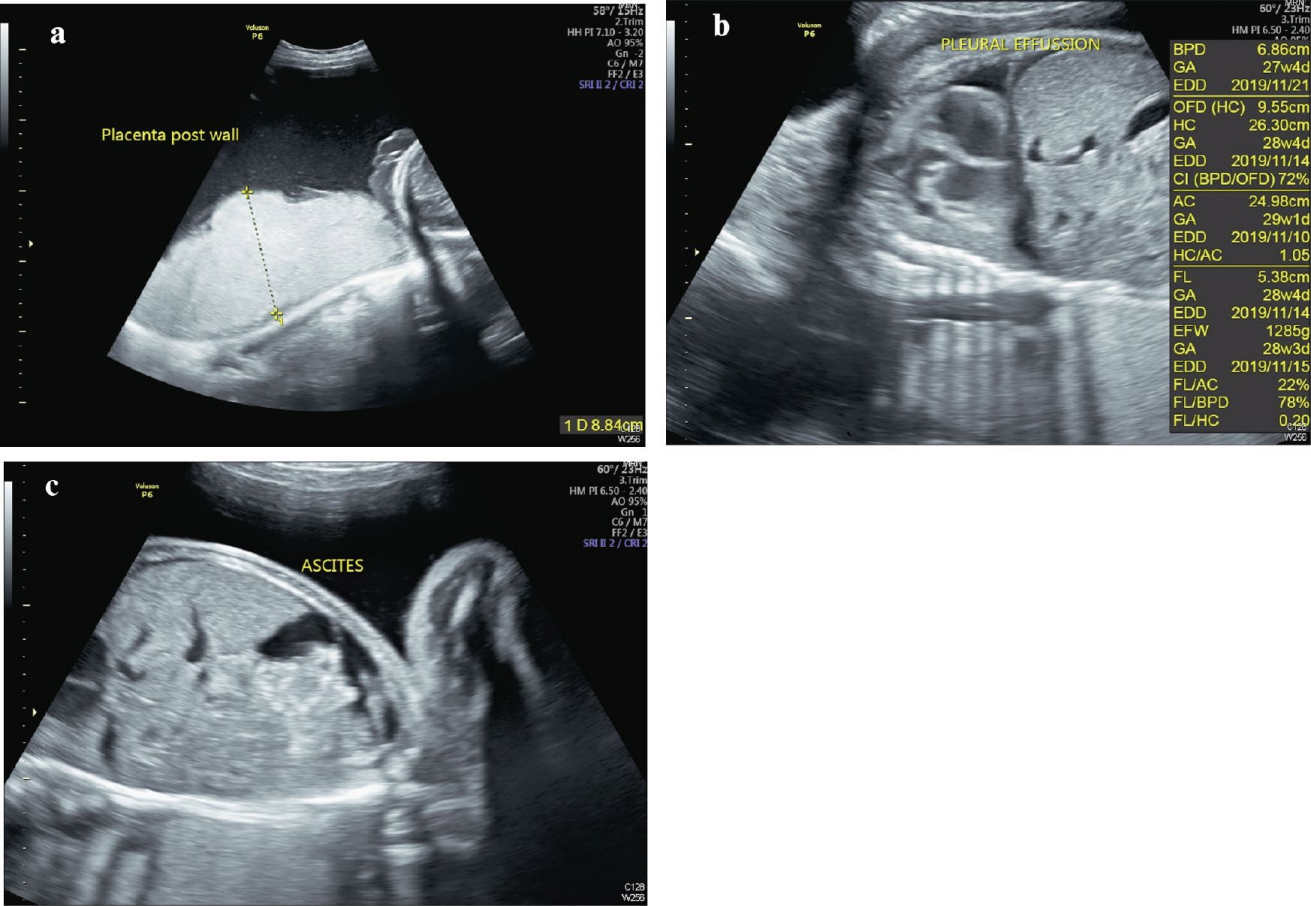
A Transabdominal sonography showing the placenta over the posterior wall of the uterine cavity with increased thickness (approximately 8.8 cm). B Transabdominal sonography showing fluid accumulation in the bilateral pleural cavity of the fetus. C Transabdominal sonography showing fluid accumulation in the abdominal cavity of the fetus. According to the findings presented in Fig. 1B and -C, hydrops fetalis was diagnosed
